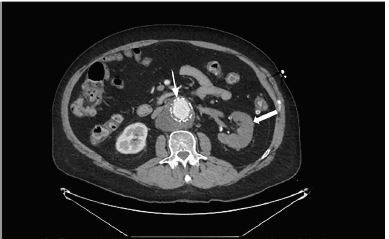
Computed tomography with contrast in axial view demonstrating thrombosed endoluminal aortic stent (thin arrow) and infarcted left kidney (thick arrow).
